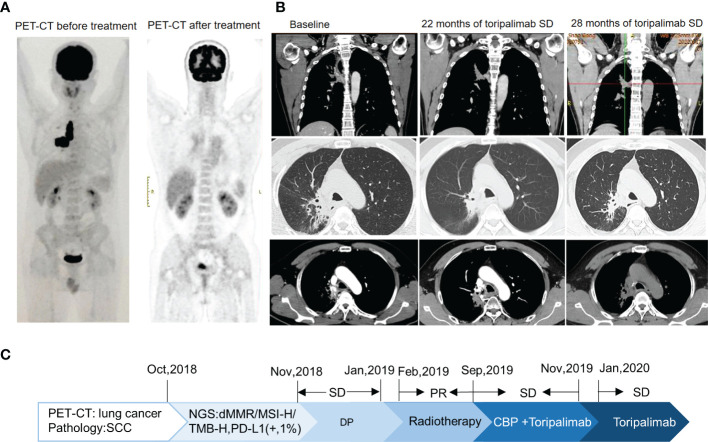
Imaging changes. (A) For PET-CT at diagnosis versus PET-CT after comprehensive treatment, the overall efficacy was PR. (B) CT images of Toripalimab during the different treatment periods. (C) The timeline of the main events of this case report.
